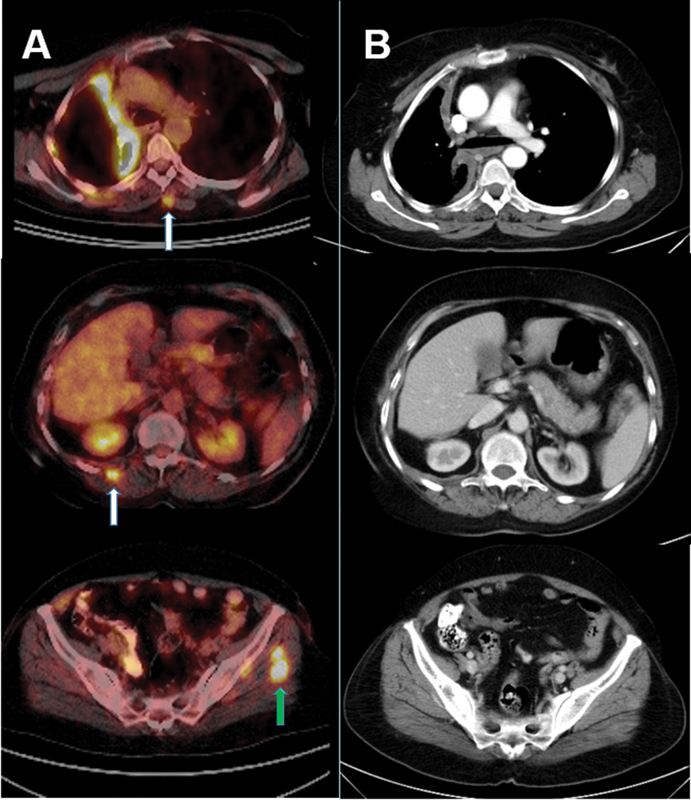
Fluorodeoxyglucose (FDG) avid metastatic lesions are clearly seen in the left and right paraspinal muscles (
